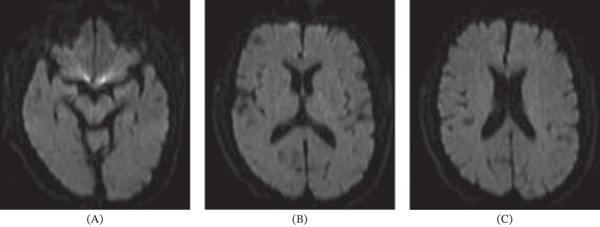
Follow‐up magnetic resonance imaging of hypoglycemic encephalopathy. Axial diffusion‐weighted imaging performed 2 months after admission shows complete resolution of hyperintensities in (A) bilateral cerebral peduncle, (B) splenium of corpus callosum, (B) posterior limb of internal capsule, and (C) corona radiata.
